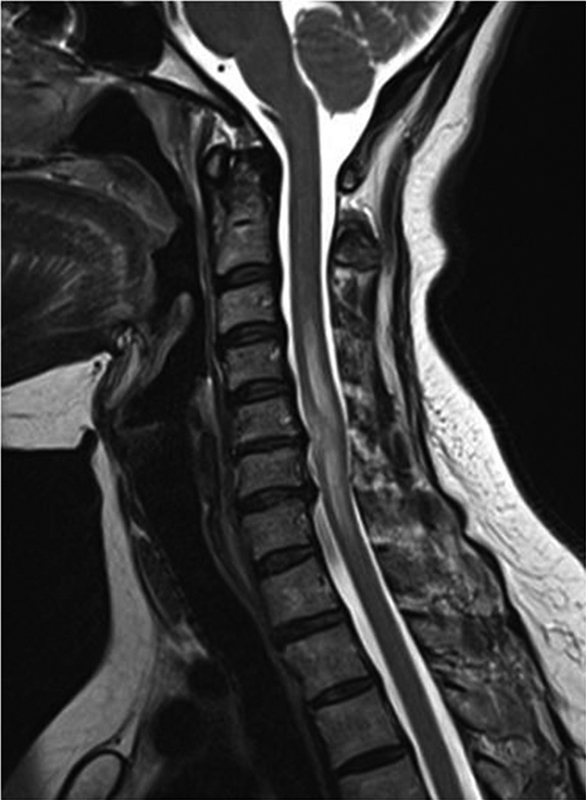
Cervical spine sag T2-weighted image showing swollen cervical cord with T2-weighted high-signal edema extending from C3 to C7 levels.
